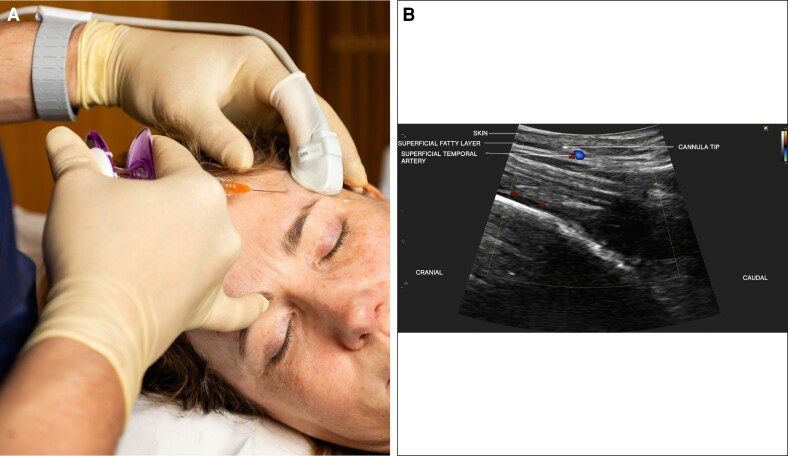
In a 57-year-old female patient, (A) the clinical view shows ultrasound-guided hyaluronic acid injection in the temple using the SibUS-In probe and a 25 G cannula introduced into the superficial fat. The probe is positioned on the index finger of the noninjecting hand, allowing simultaneous palpation and stable control during injection. (B) Doppler ultrasound imaging visualizes the 25 G cannula tip using an out-of-plane approach, in close relation to the superficial temporal artery, with both the superficial and deep temporal arteries clearly identified.
